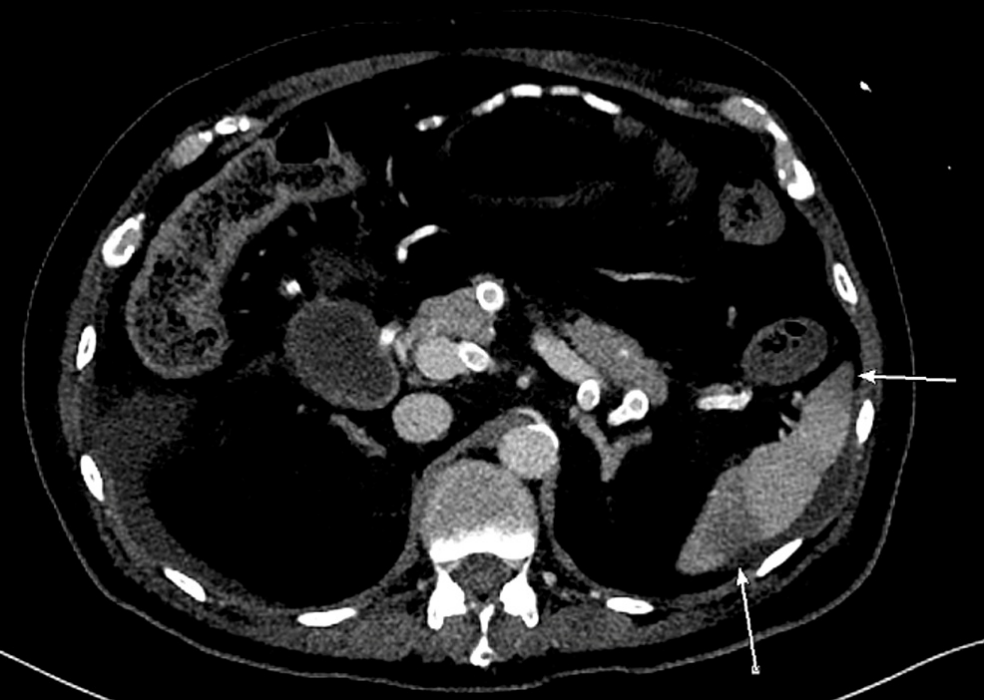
An axial plane image of the CT abdomen and pelvis showing the multiple splenic infarctions as demonstrated by the arrows and hypodensities within the spleen.
